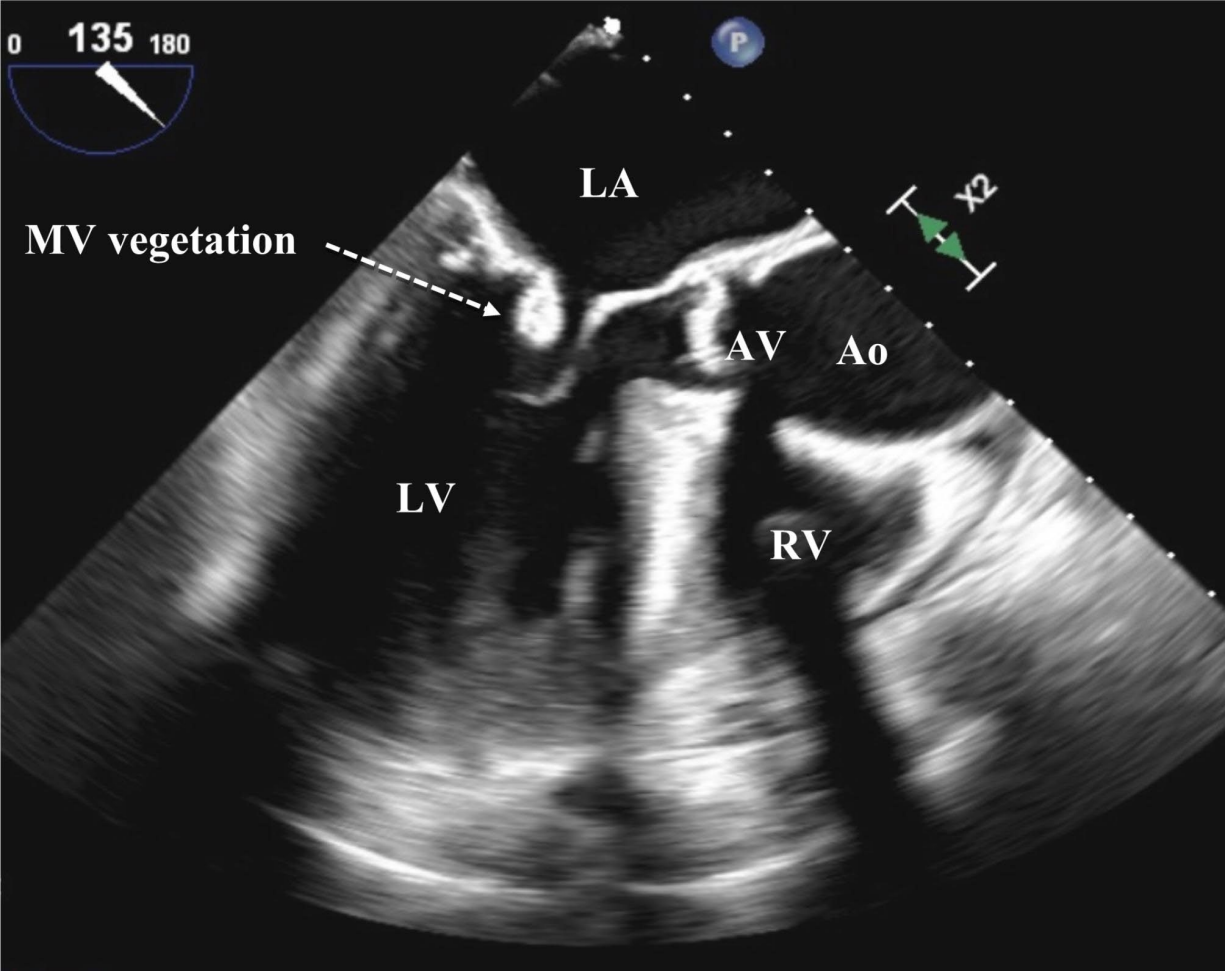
Transesophageal echocardiogram with mid-position view, 135° left: mitral valve with a vegetation of the posterior leaflet. Abbreviations: Ao, ascending aorta; AV, aortic valve; LA, left atrium; LV, left ventricle, MV, mitral valve; RV, right ventricle
